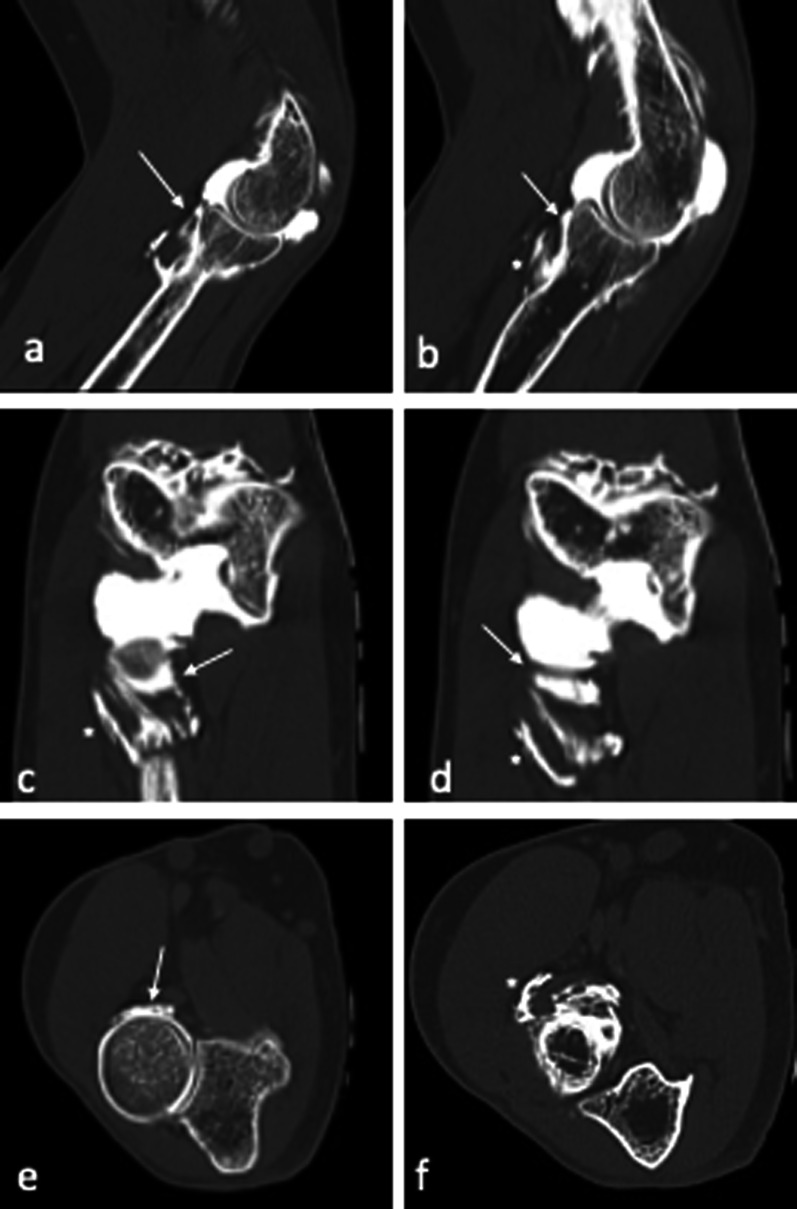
Symptomatic minor instability of the lateral elbow (SMILE) with a high-degree lesion of the annular ligament (AL) of the LCL complex. Consecutive sagittal (a, b), coronal (c, d) and axial (e, f) CT-arthrography scans highlight the ruptured AL (white arrows) and display extra-articular leakage (asterisks) through the damaged articular capsule
